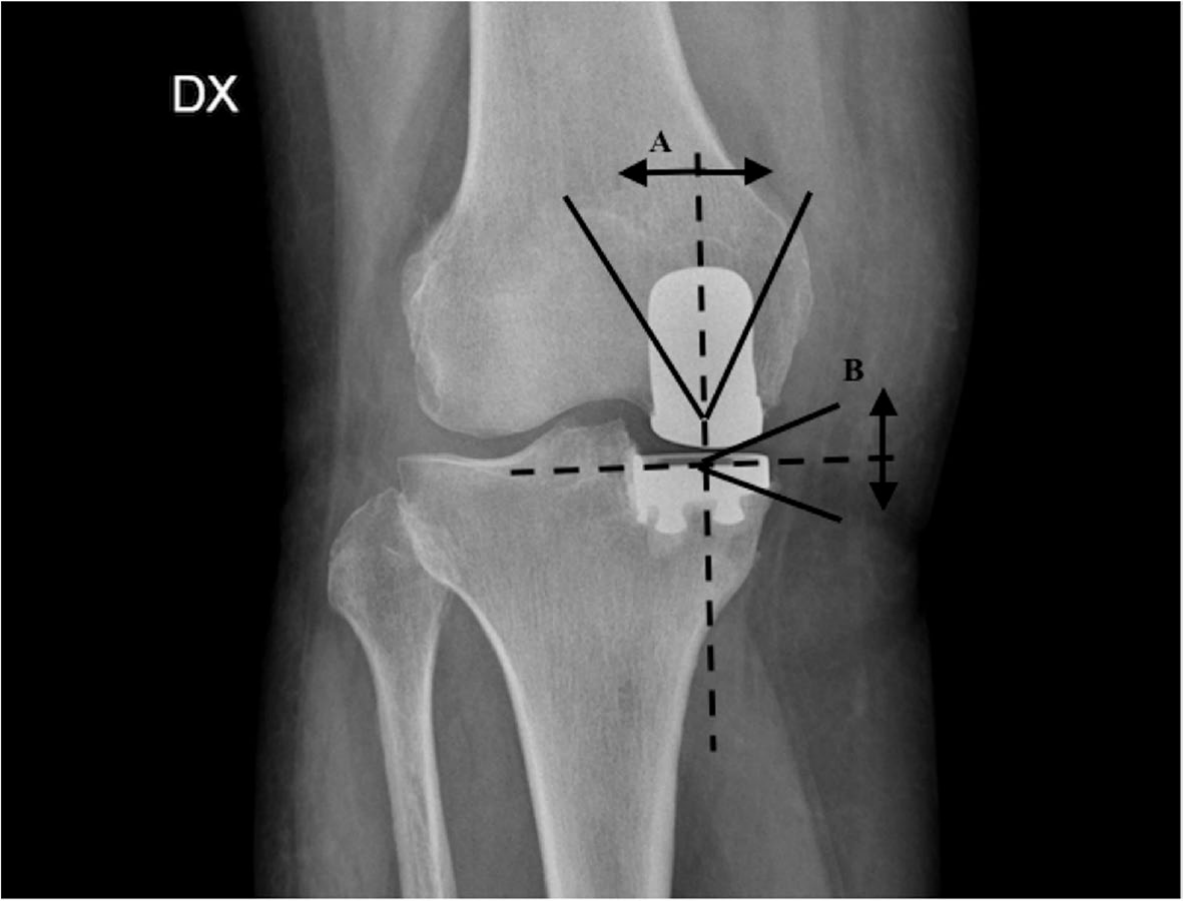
Antero-posterior radiograph, right knee; Radiological parameters measured: femoral varus/valgus angle (A); tibial varus/valgus angle (B)
